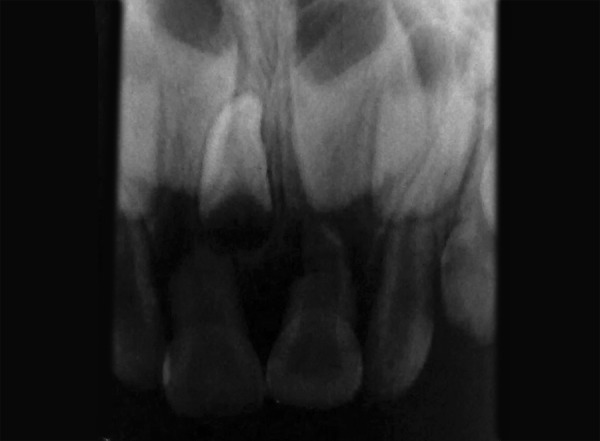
Intraoral periapical radiograph revealing inverted impacted supernumerary tooth (case III)
